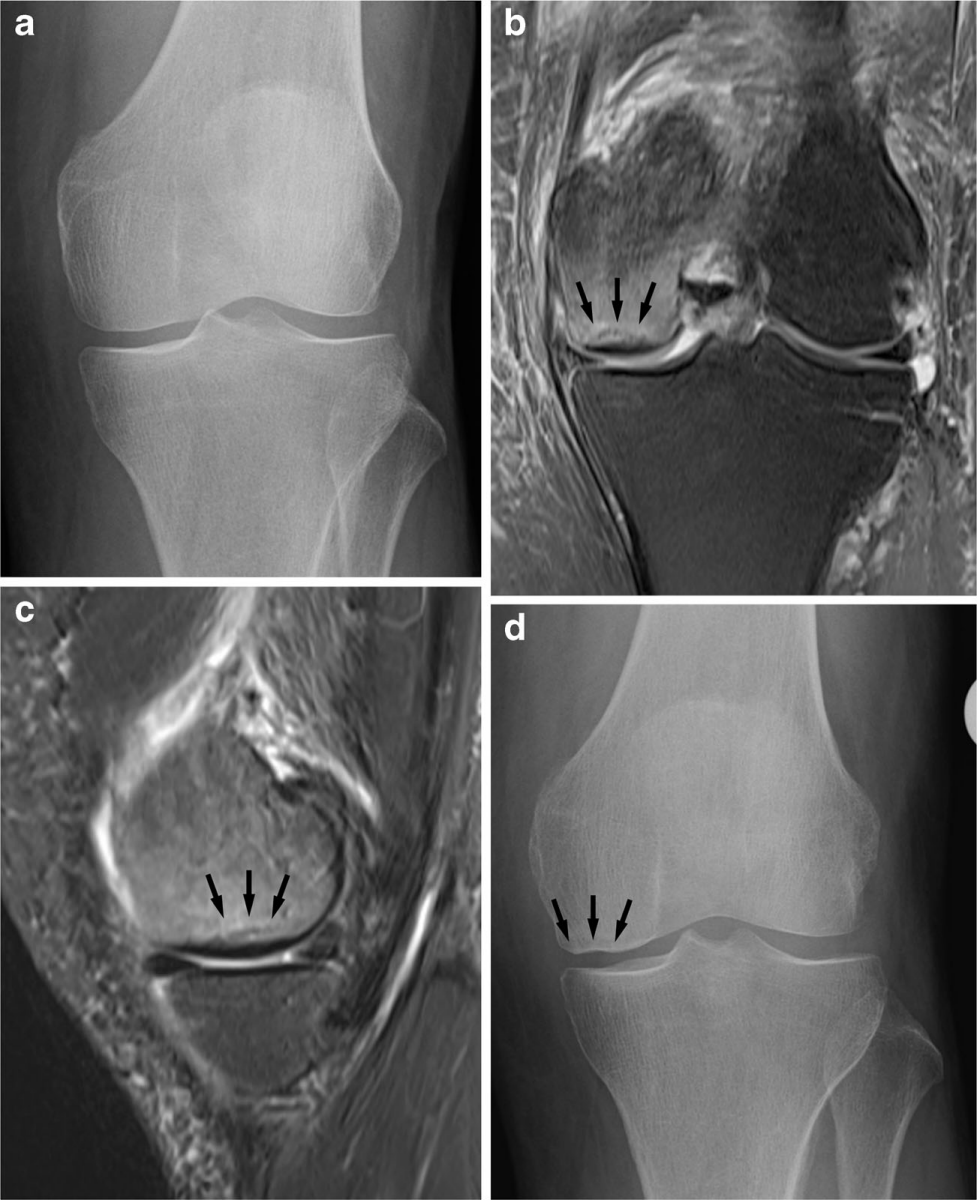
An elderly female with sudden spontaneous onset of pain. Initial radiographs (shown AP view, a) do not show a focal anomaly. MRI 1 month later performed for persistent pain shows extensive bone marrow oedema-like change in the medial femoral condyle with a subchondral sclerotic area consistent with an insufficiency fracture (b, c; PD-FS; see arrows). The features are those of spontaneous osteonecrosis of the knee, SONK. Note the degeneration/tear of the medial meniscus, a common association of SONK. Radiographic follow-up 1 month after the MRI shows partial cortical collapse of the medial femoral condyle (d, see arrows)
